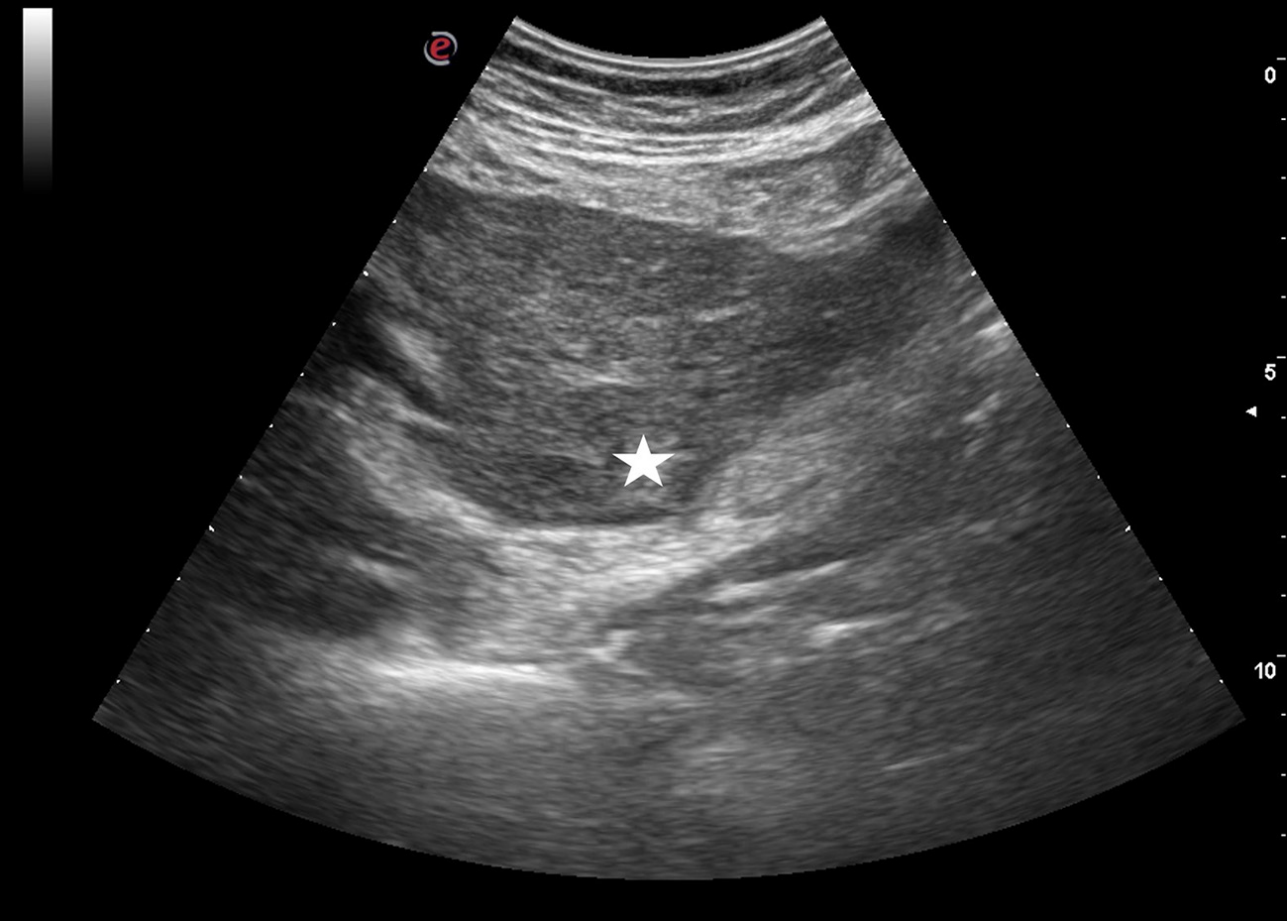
Ultrasound image of a 62-year-old male patient. A focal lesion (asterisk) is observed in the heterogeneous and coarse granular liver parenchyma of the patient who is being followed up for hepatitis B.
